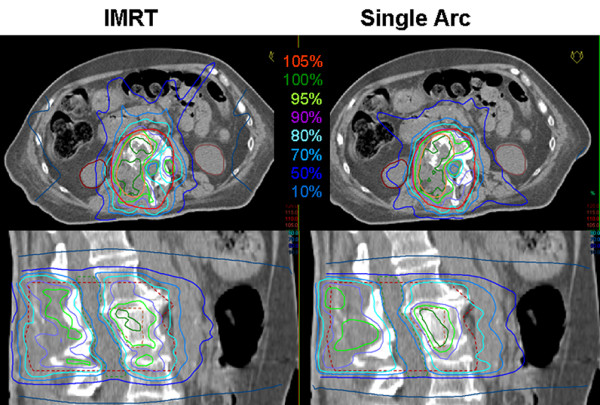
Dose distributions for metastases in the lumbar vertebra. Comparison of dose distributions achieved with 7-field IMRT (left) and Single Arc VMAT (right) on representative transversal (top) and sagittal (bottom) CT slices. The PTV is drawn in red, the GTV in orange, the spinal cord in green, and the kidneys in maroon. Isodose lines are shown in percent of the prescription dose, i.e. 55 Gy to the average of the GTV.
